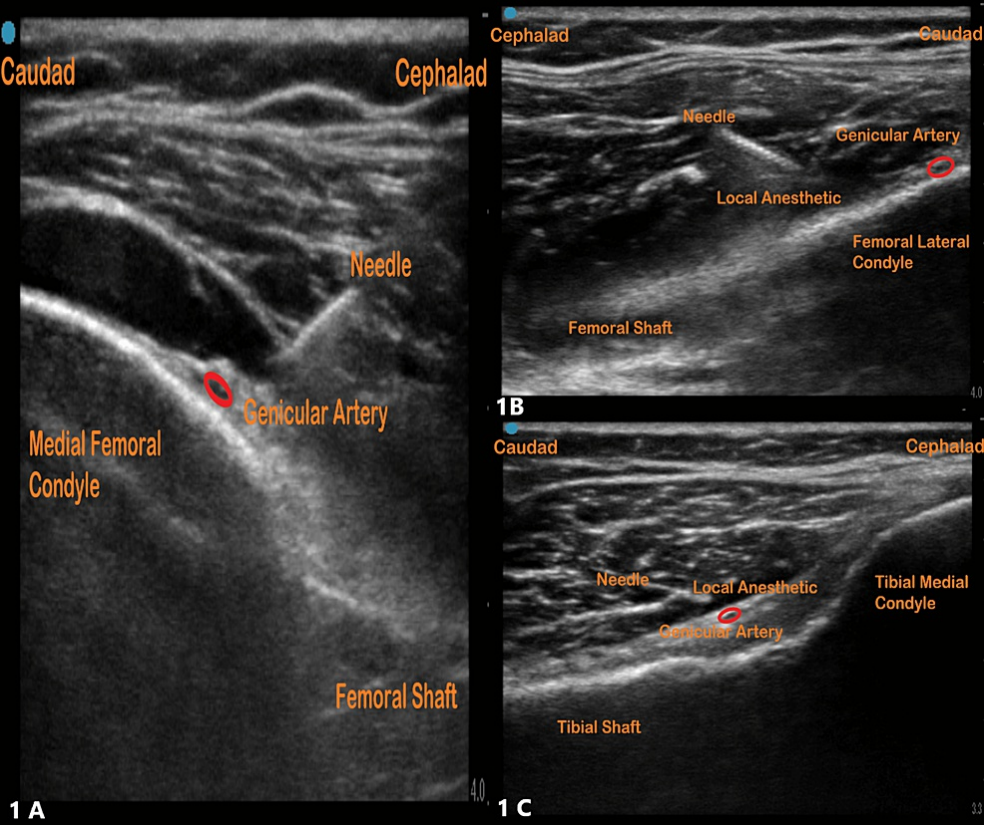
Ultrasound images of genicular nerve blocks. 1A: Superomedial genicular nerve block. 1B: Superolateral genicular nerve block. 1C: Inferomedial genicular nerve block.
